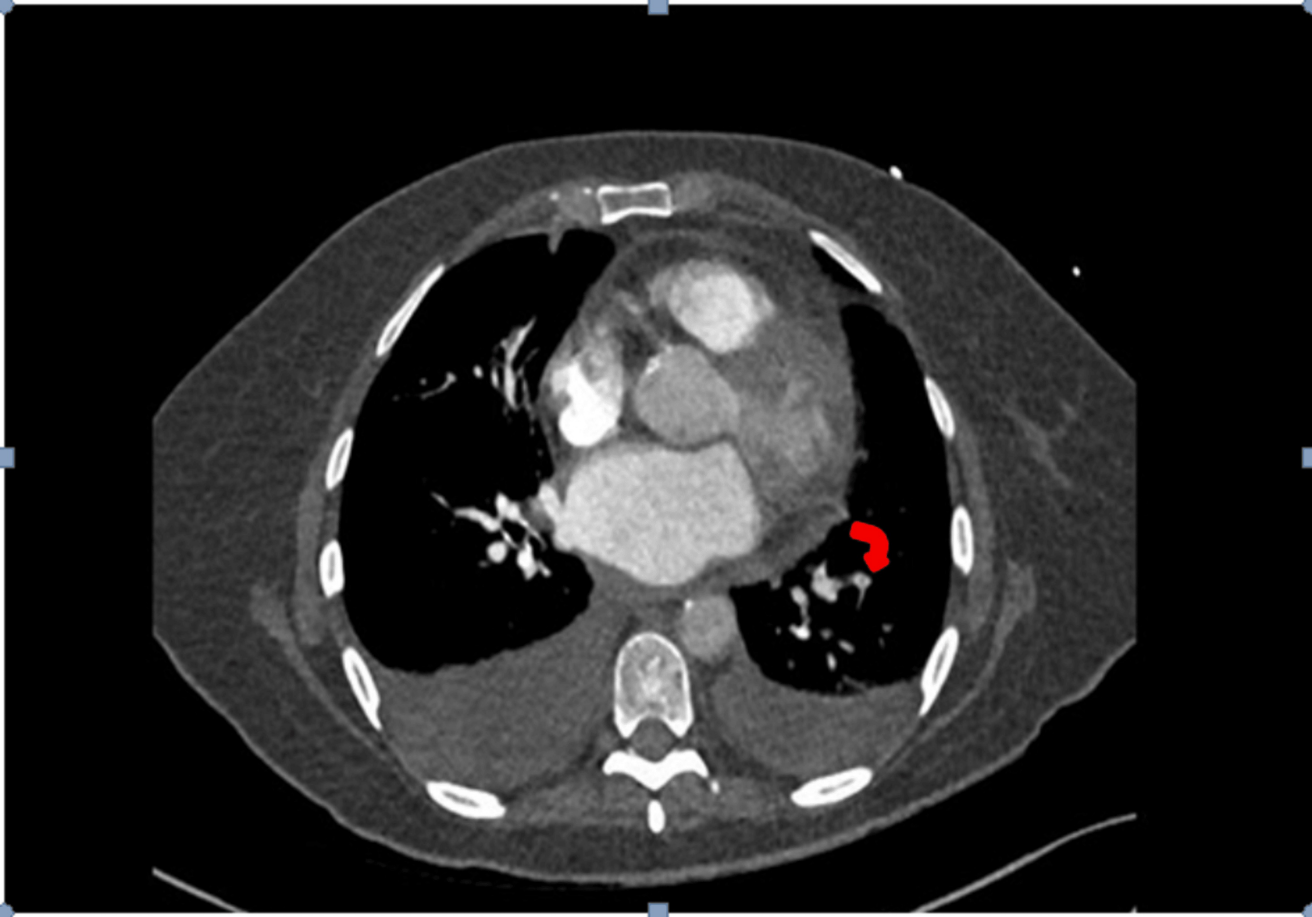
CT pulmonary angiogram showing segmental pulmonary emboli (red arrow)
